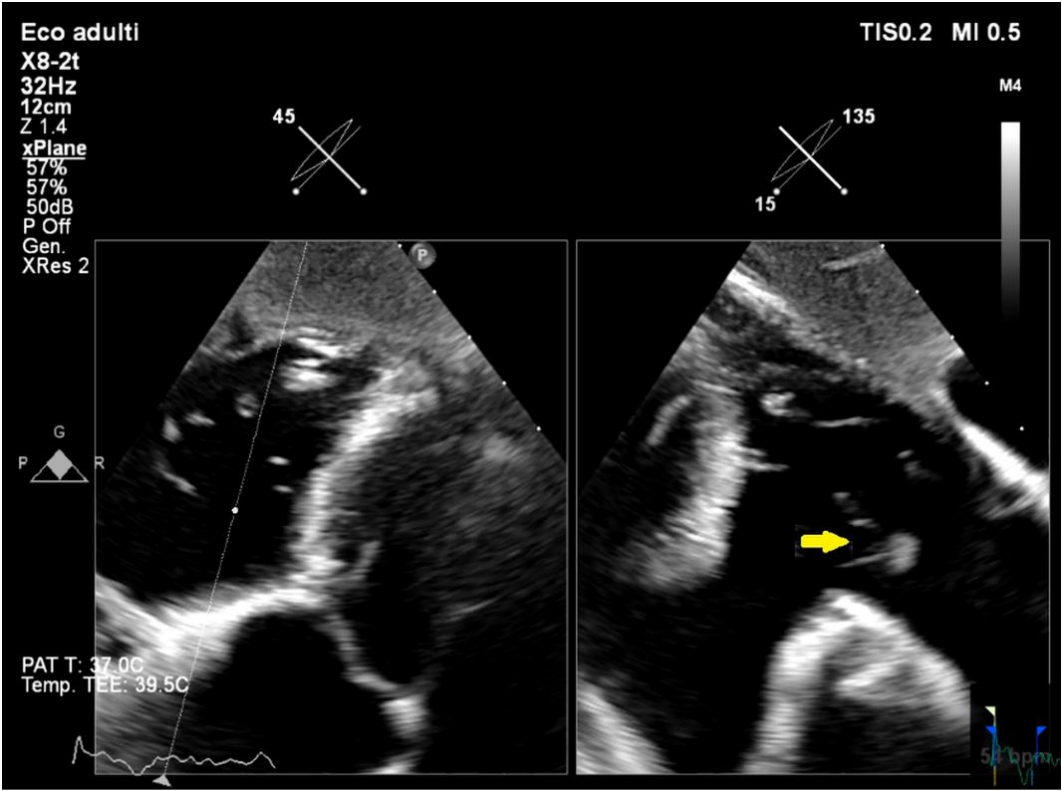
Off-axis short axis view of the tricuspid valve from the transoesophageal transgastric window with X-plane imaging, showing a ‘finger-in-glove’ and ‘garland-like’ appearance with adherent filaments resembling second-order chordae tendineae.
